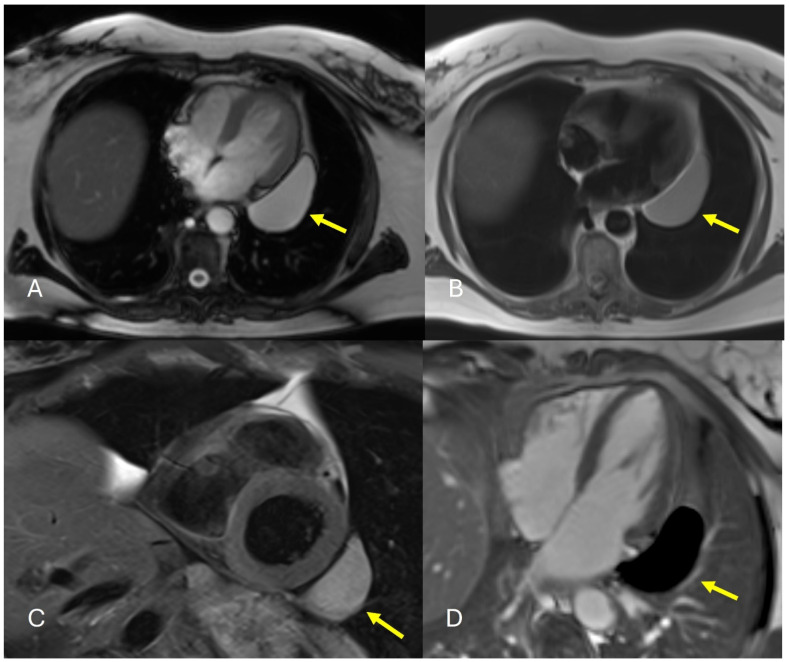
(A–D): Cardiac Magnetic Resonance imaging on the same patient (performed a few years later) depicting interval increase in size of the well-defined lesion along the left lateral heart border (yellow arrow), which appears hyperintense on axial white blood (A), axial black blood (B), T2 fat sat short axis (C) images with no evidence of internal or peripheral enhancement on post contrast images (D), consistent with pericardial cyst.
